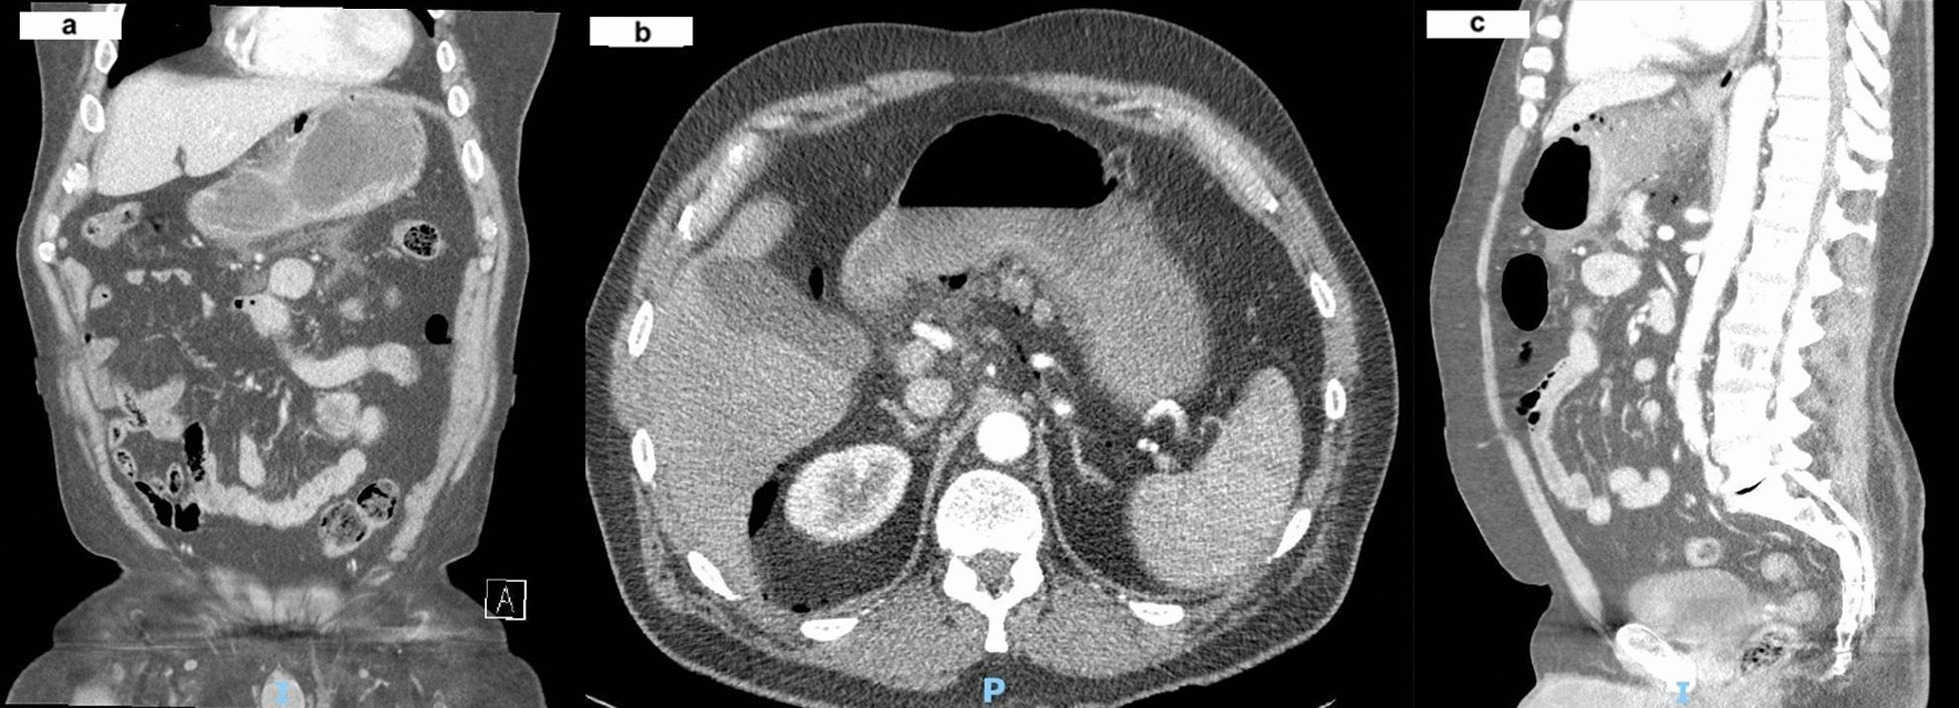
a Axial slice of abdomen CT showing free air in peritoneal cavity. b Coronal slice of abdomen CT showing free air near the lesser curvature. c Sagittal slice of abdomen CT showing subhepatic free air
